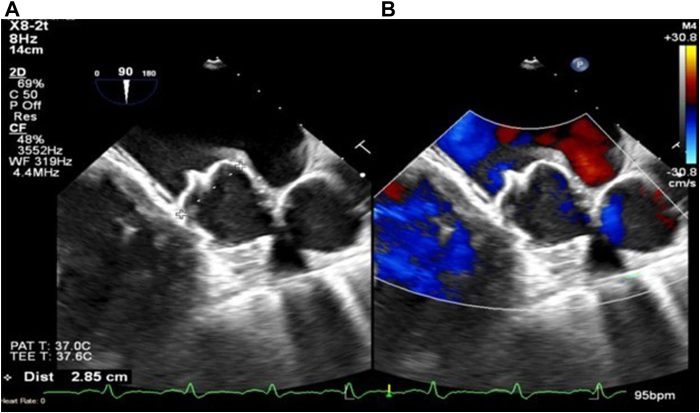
Intraprocedural TEE during the WATCHMAN FLX Pro device implant. 90° TEE showing 2D (A) and color Doppler images (B) demonstrating no peridevice leak after the release of the 40 mm WATCHMAN FLX Pro device. 2D = 2-dimensional; TEE = transesophageal echocardiogram.
